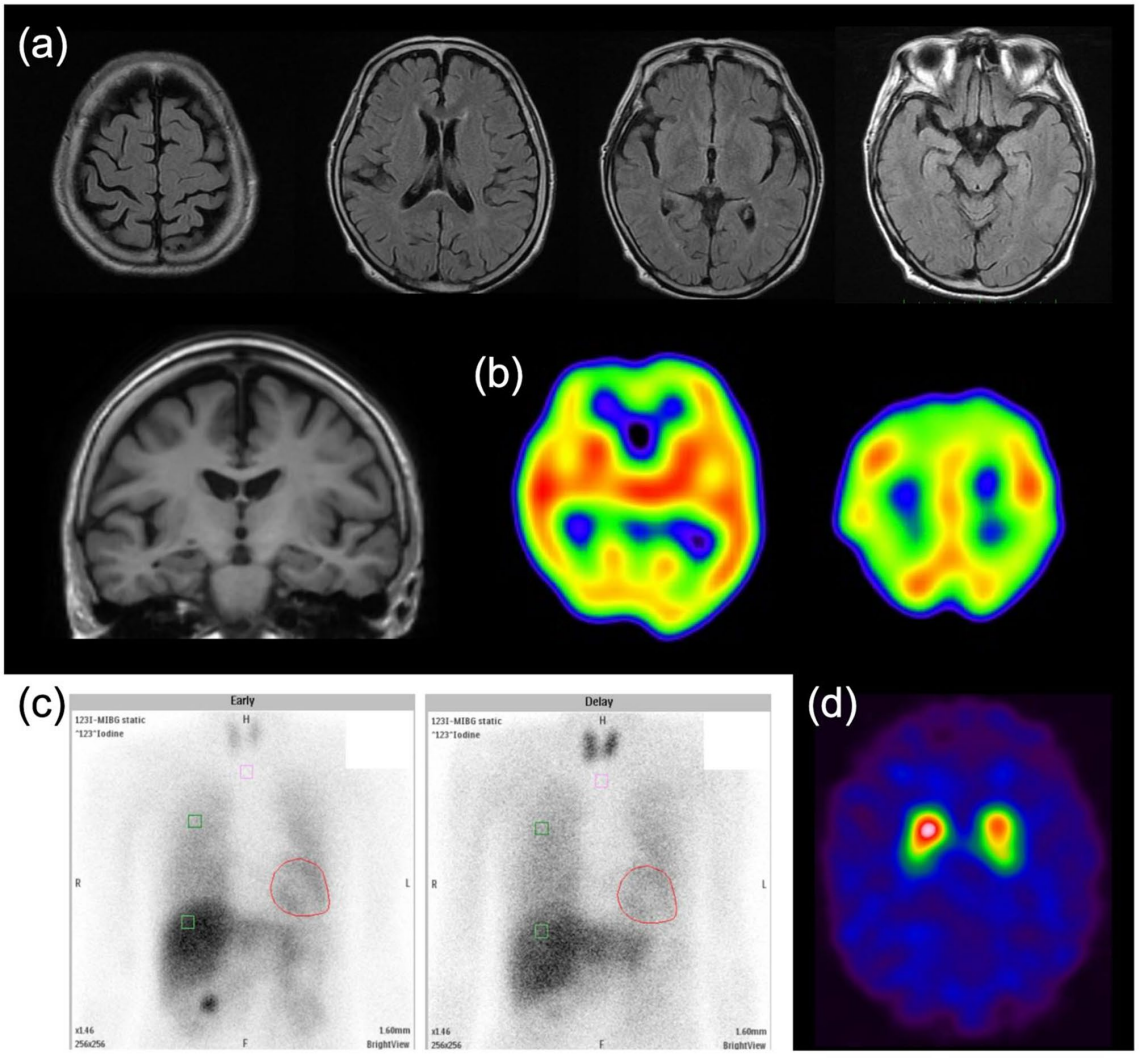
Neuroimaging findings in this patient with dementia with Lewy bodies. (a) Cranial magnetic resonance imaging showed no abnormalities. (b) N-isopropyl-p-iodoamphetamine single-photon emission computed tomography showed reduced perfusion in the bilateral parietal, frontal, precuneus, and occipital lobes. (c) ^123^I-meta-iodobenzylguanidine myocardial scintigraphy showed that the heart-to-mediastinum ratio was maintained at 3.69 and 2.41 in the early and late stages, respectively, but the washout rate was high at 59.5%, suggesting mild abnormality of cardiac sympathetic nerve function. (d) ^123^I-N-omega-fluoropropyl-2-betacarbomethoxy-3-beta (4-iodophenyl) nortropane dopamine-transporter single-photon emission computed tomography showed decreased uptake in the posterior striatum, especially on the left side
